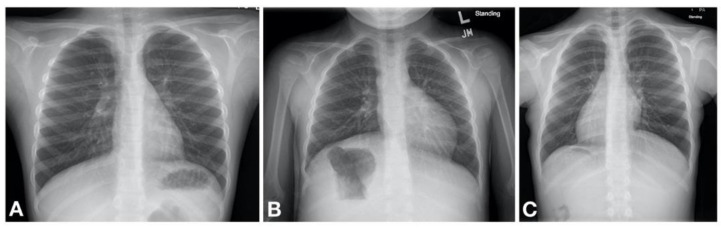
Machogu et al. Laterality defects found on chest radiography of patients with PCD. (A) Normal; Left sided cardiac apex with left sided stomach and right sided liver (B) Situs Ambiguous; Left sided cardiac apex with right sided stomach and left sided liver (C) Situs Inversus Totalis; right sided cardiac apex with right sided stomach and left sided liver.
